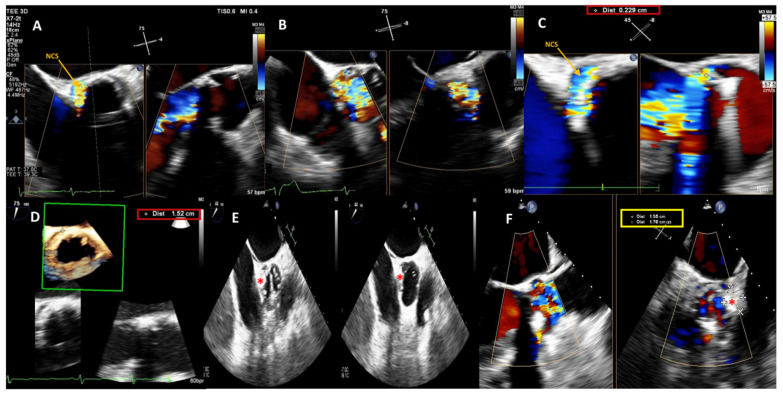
Baseline 2D/3D Transesophageal Echocardiogram (TEE) color Doppler showing a 15 × 2.3 mm large crescent-shaped NCS paravalvular leak with severe regurgitant jet (A–C) and 3D TEE color Doppler showing the PVL dimensions and location (D). Post-procedure 2D TEE color Doppler showing the correct position of the device (red star) during the cardiac cycle (diastolic and systolic frames, respectively) (E) and confirming stability of the 14 × 6 mm rectangular waist PLD (red star) without impingement on the mechanical prosthetic aortic valve (F). NCS, non-coronary sinus; PLD, Occlutech Paravalvular Leak device.
